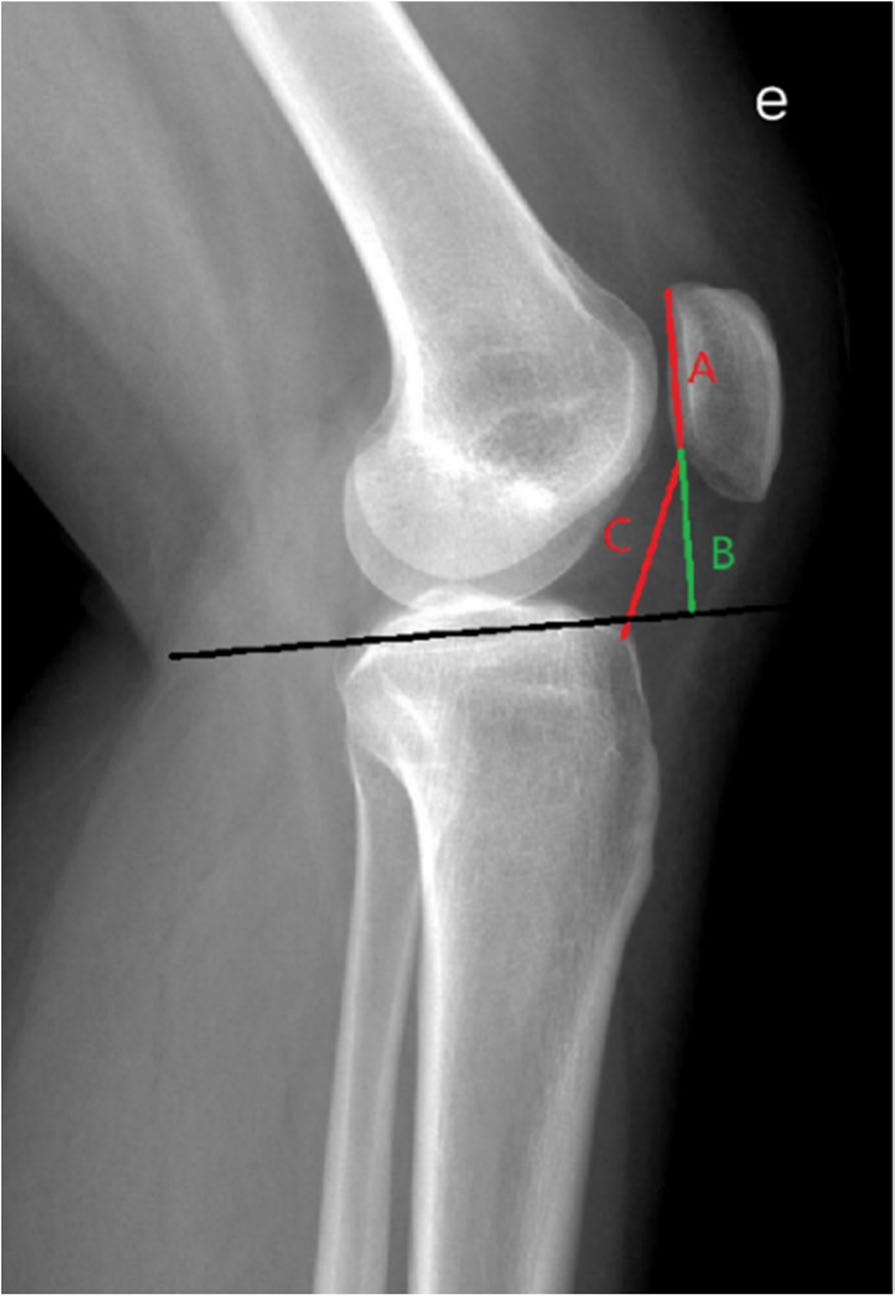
Radiographic patellar height indices. Caton-Deschamps index, The ratio of the distance from the lowest point of the patellar articular surface to the upper corner of the tibial plateau (C) to the length of the patellar articular surface (A). Blackburne-Peel index, The ratio of the vertical distance (B) from the lowest point of the patellar articular surface to the tibial plateau to the length of the patellar articular surface (A)
